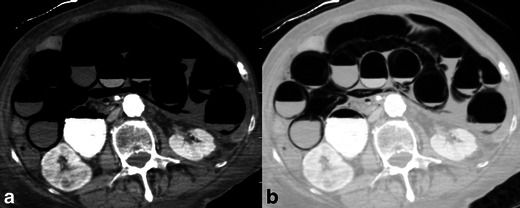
Pneumatosis intestinalis. Axial contrast-enhanced CT images in a soft tissue and b lung windows demonstrate extensive pneumatosis intestinalis secondary to superior mesenteric arterial occlusion. Note the increased conspicuity of pneumatosis with the use of lung windows
